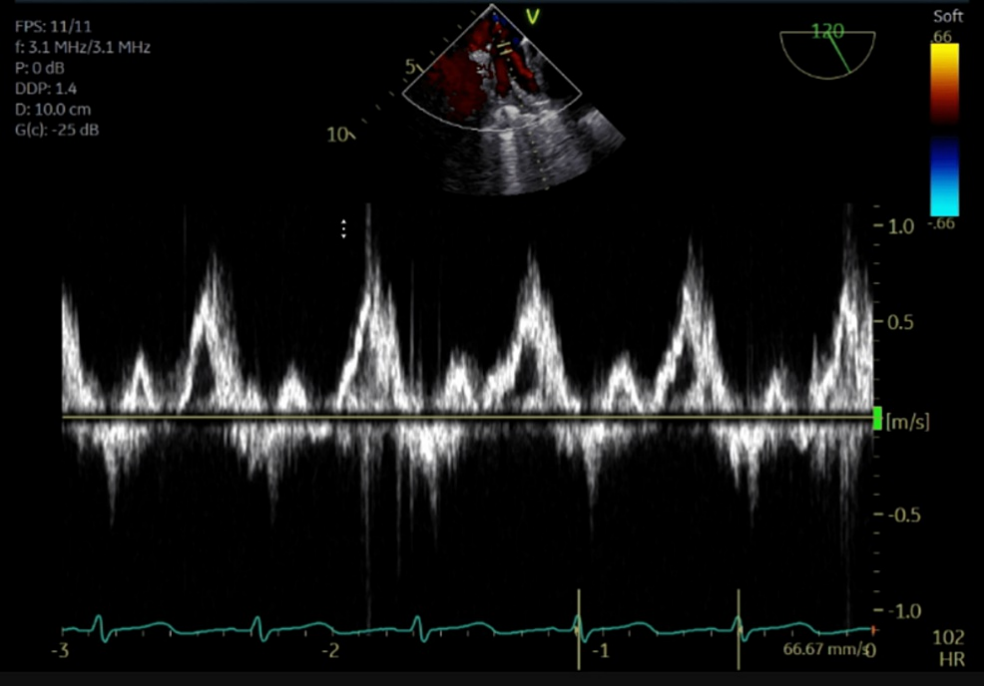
Pulsed-wave Doppler of the left superior pulmonary vein showing systolic flow reversal.
